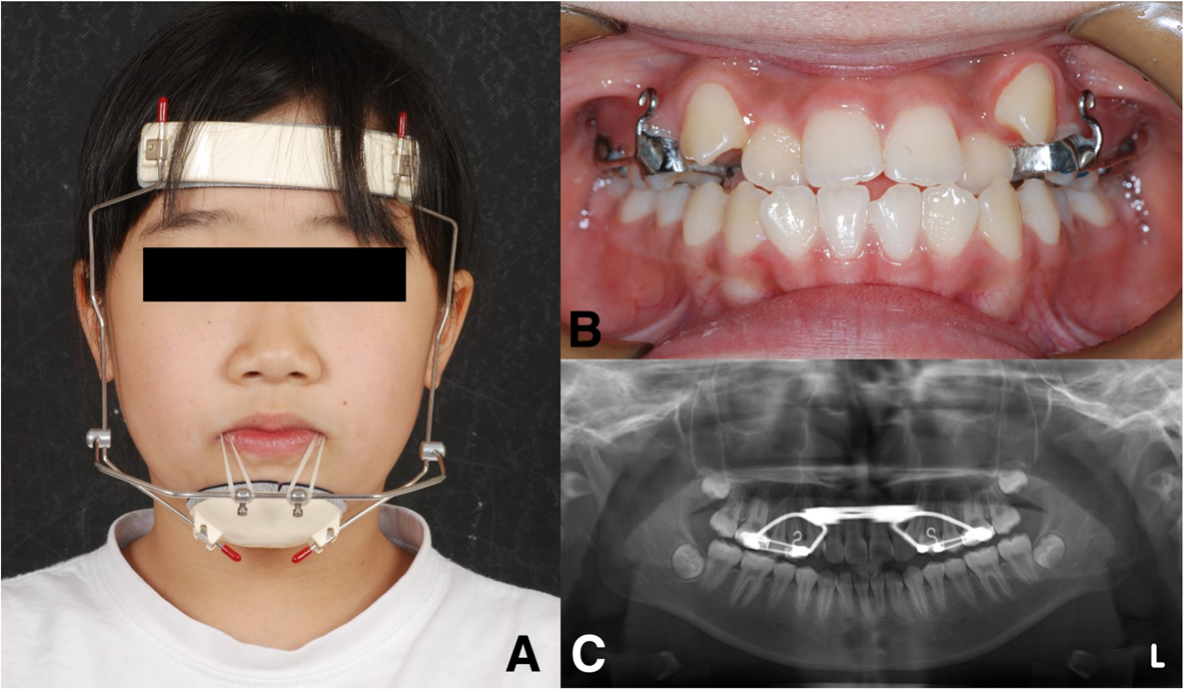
a Frontal view of the patient with a facemask. b Intraoral view of face mask and rapid palatal expansion. c Panoramic radiograph showing rapid palatal expansion
